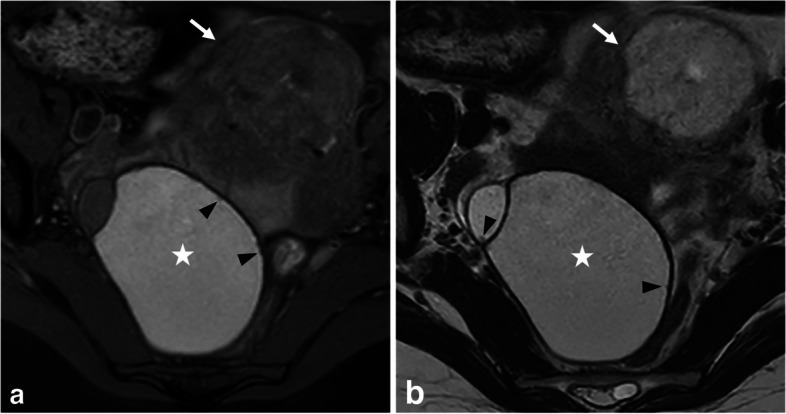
Right-sided paratubal serous papillary cystadenofibroma in a 29-year-old woman with right pelvic pain during first trimester pregnancy. a Axial T1-W fat-suppressed and (b) axial T2-W MR images show a bilocular paratubal cystic lesion (star) with a T1- and T2-hyperintense posterior loculus (star). The wall is irregular with endocystic tiny papillary projections (arrowheads). Note the gravid uterus (arrows)
